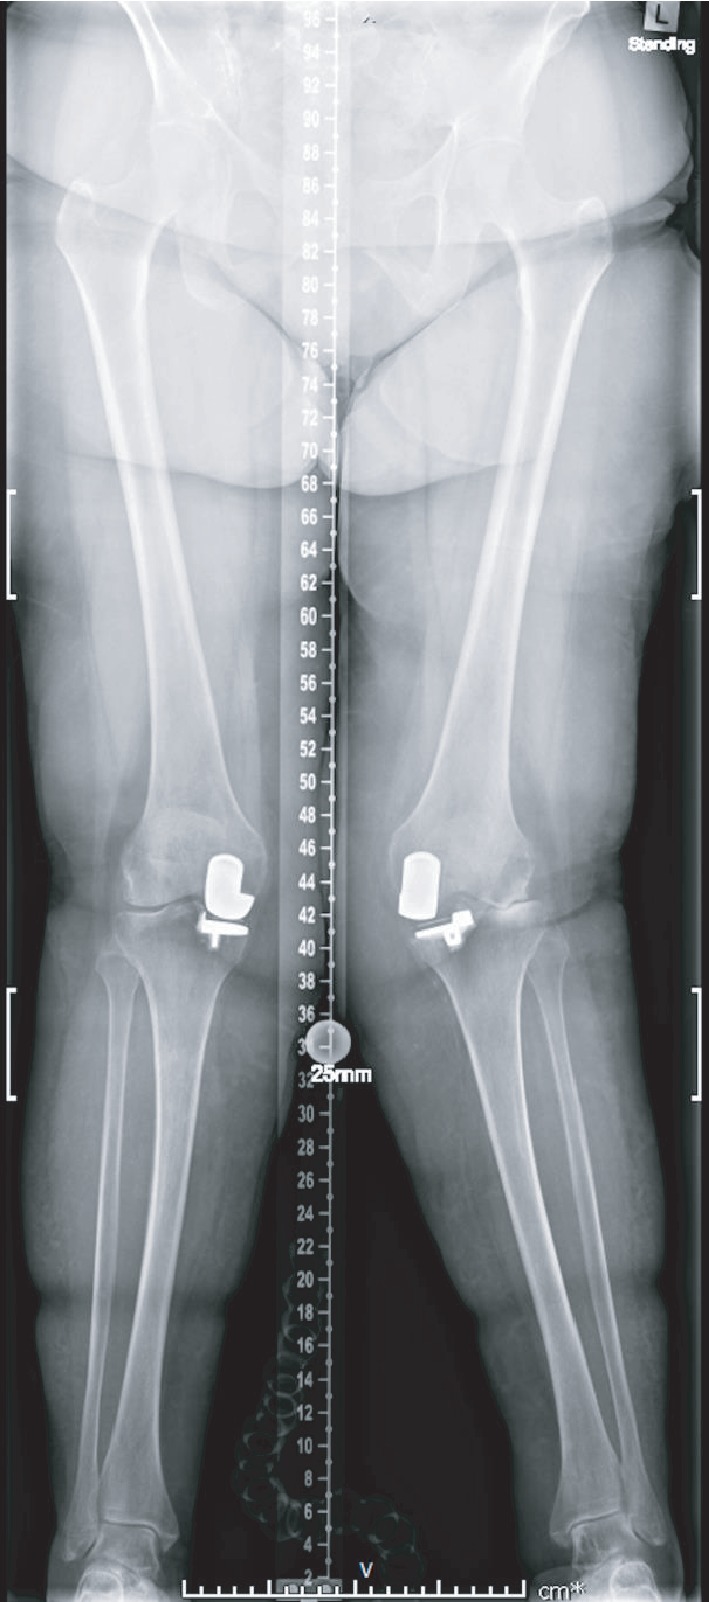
Long-leg radiographs demonstrating bilateral medial unicompartmental knee replacements. Valgus deformity of the left knee with loss of lateral joint space.
